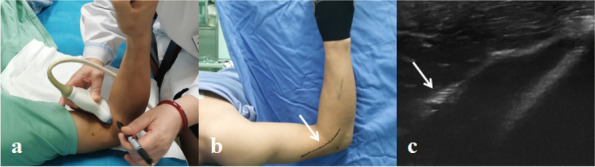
A 33-year-old male in the preoperative ultrasound group. a prior subcutaneous radial nerve demonstrating the transducer position and marking (dots) of the radial nerve during mapping; (b) the path of the radial nerve (white arrow) was drawn by a solid line; (c) the ultrasound images of the radial nerve (white arrow)
